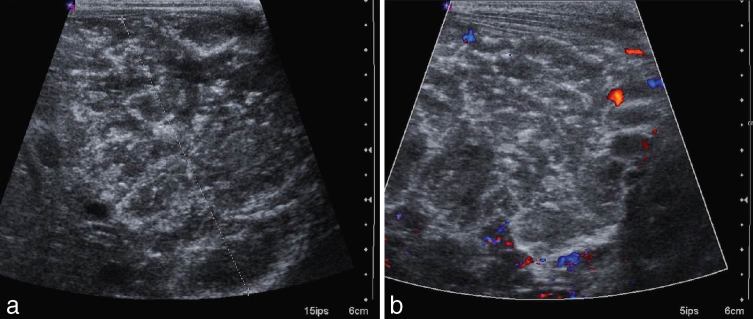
Ultrasound demonstrated a hyperechoic, heterogeneous hepatic mass (between calipers) (a) with moderate vascularization on Doppler ultrasound (b) and small calcifications.
